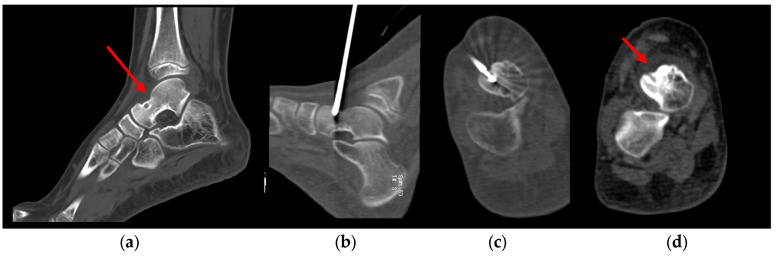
Pre-treatment CT image (a) of an osteoid osteoma in the talus in a 12 years old child with a persistent limp for 8 months; (b) sagittal and (c) axial CT image during cryoablation procedure using an IceSeed cryoprobe; (d) 1-month post-procedure CT image.
